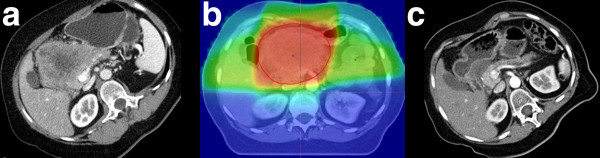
CT scan of a female patient before (A) and after (C) neoadjuvant CRT showing a partial remission of the pancreatic tumour. Dose distribution of the applied treatment plan (B).
